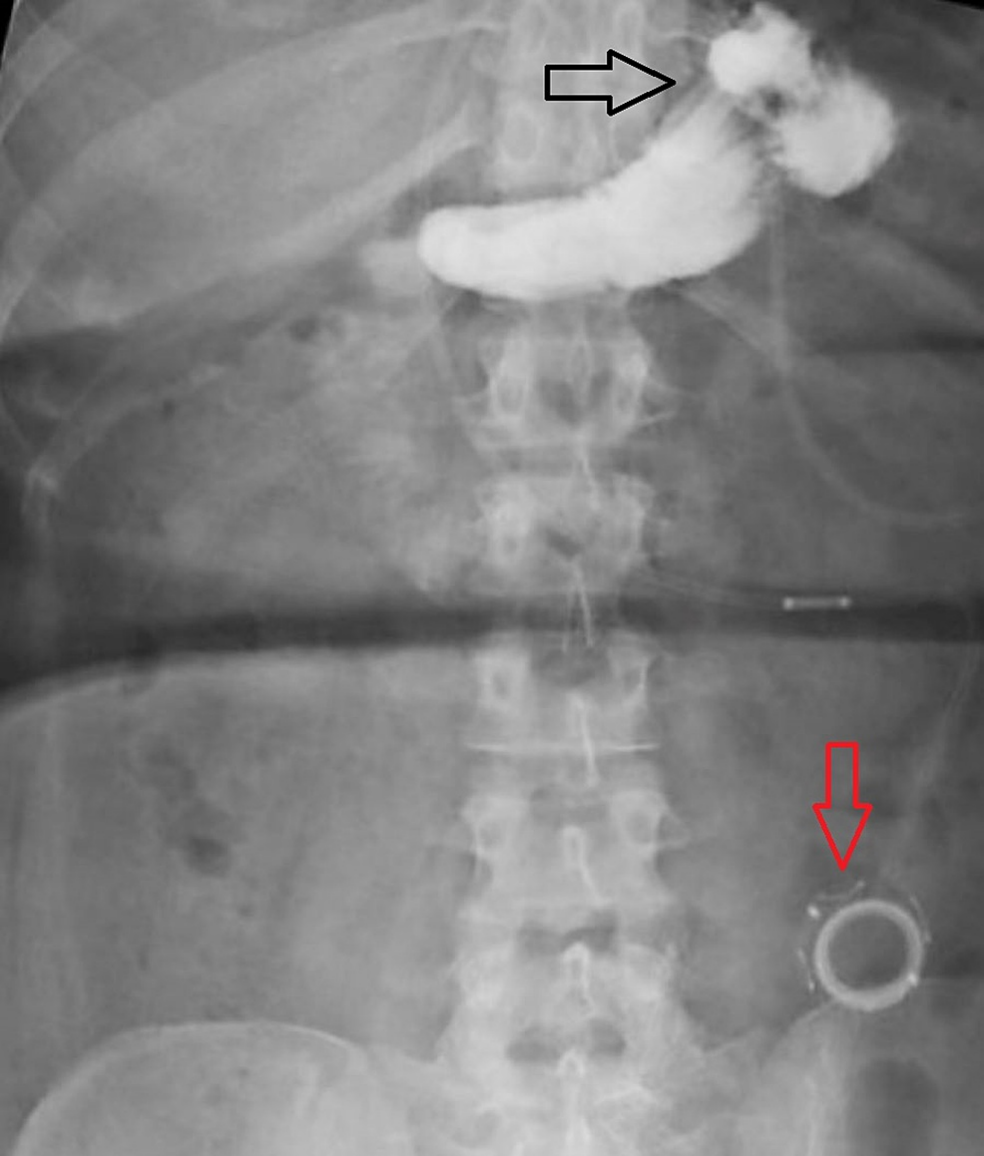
Abdominal radiograph (KUB) showing gastric band (black arrow) and port placement (red arrow).
